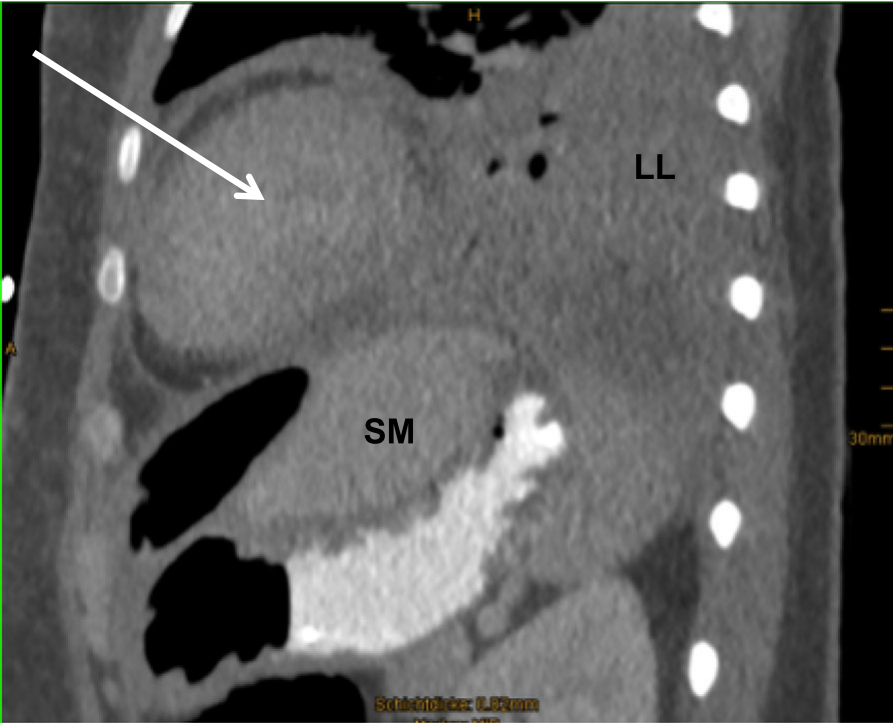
CT-scan demonstrating left subphrenic abscess with destruction of the diaphragm and development of pneumonia per continuitatem. LL, left lower lobe; SM, stomach; white asterisk: subphrenic abscess
